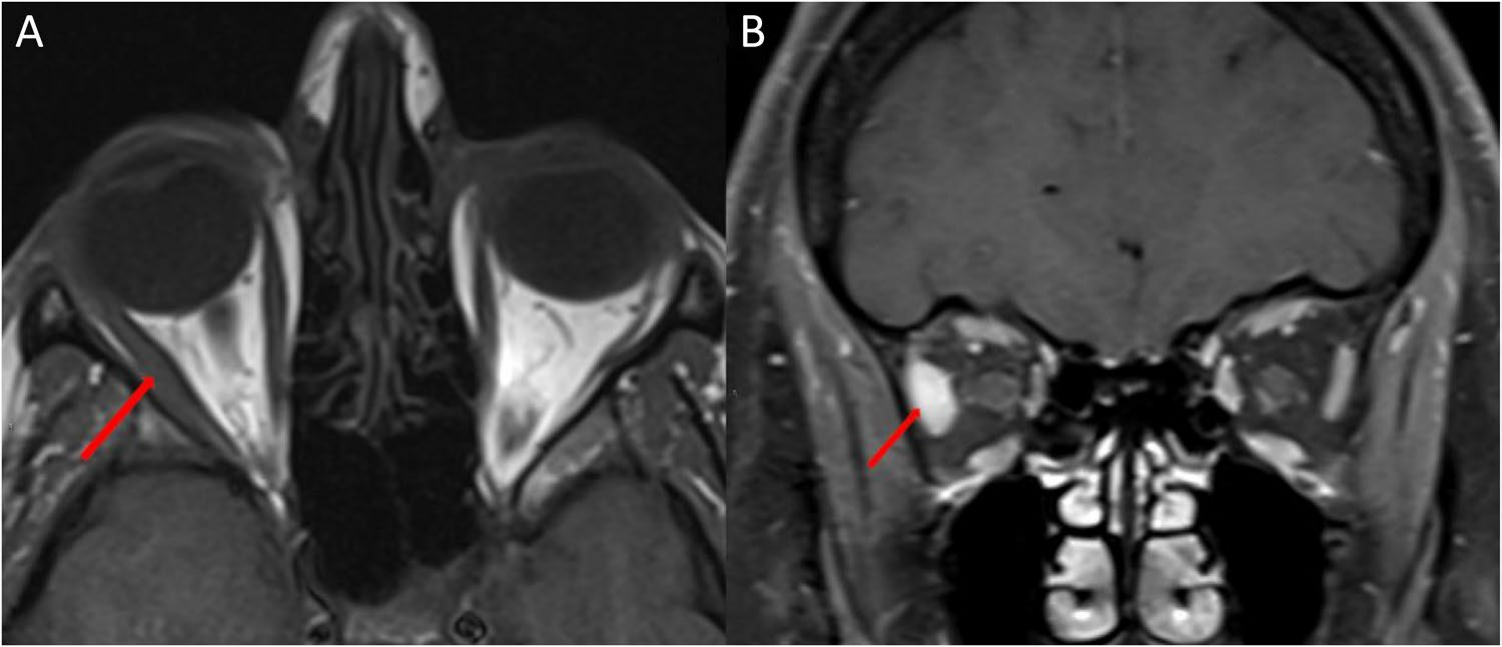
Idiopathic orbital myositis. Axial T1-weighted MRI (A) showing isointense enlargement of the right lateral rectus muscle including the anterior tendon (arrow). Coronal fat-suppressed contrast-enhanced T1-weighted MRI (B) showing enlargement and enhancement of the right lateral rectus muscle
